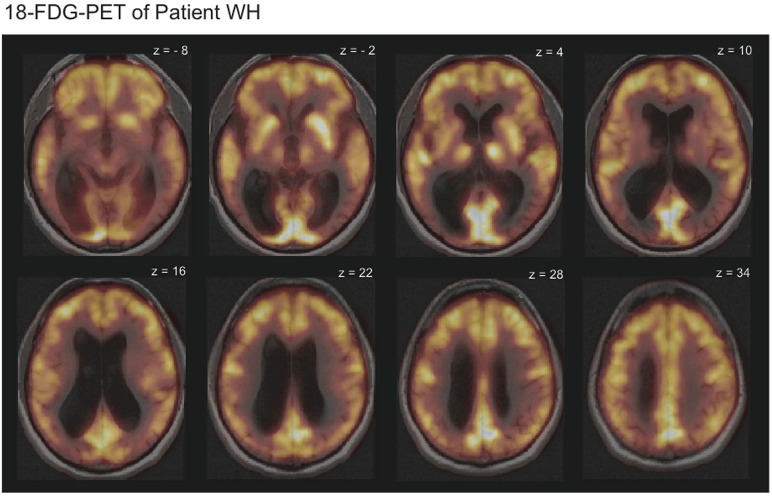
18-Fluorodeoxyglucose positron emission tomography (FDG-PET-CT) scans overlaid with the magnetic resonance imaging (MRI) scans revealed reduced metabolism in the temporo-occipital cortex bilaterally for patient WH. Displayed is HW's 18-FDG-uptake in comparison to the average of 20 healthy subjects. Bright yellow colors indicate high and dark red colors low 18-FDG-uptake.
